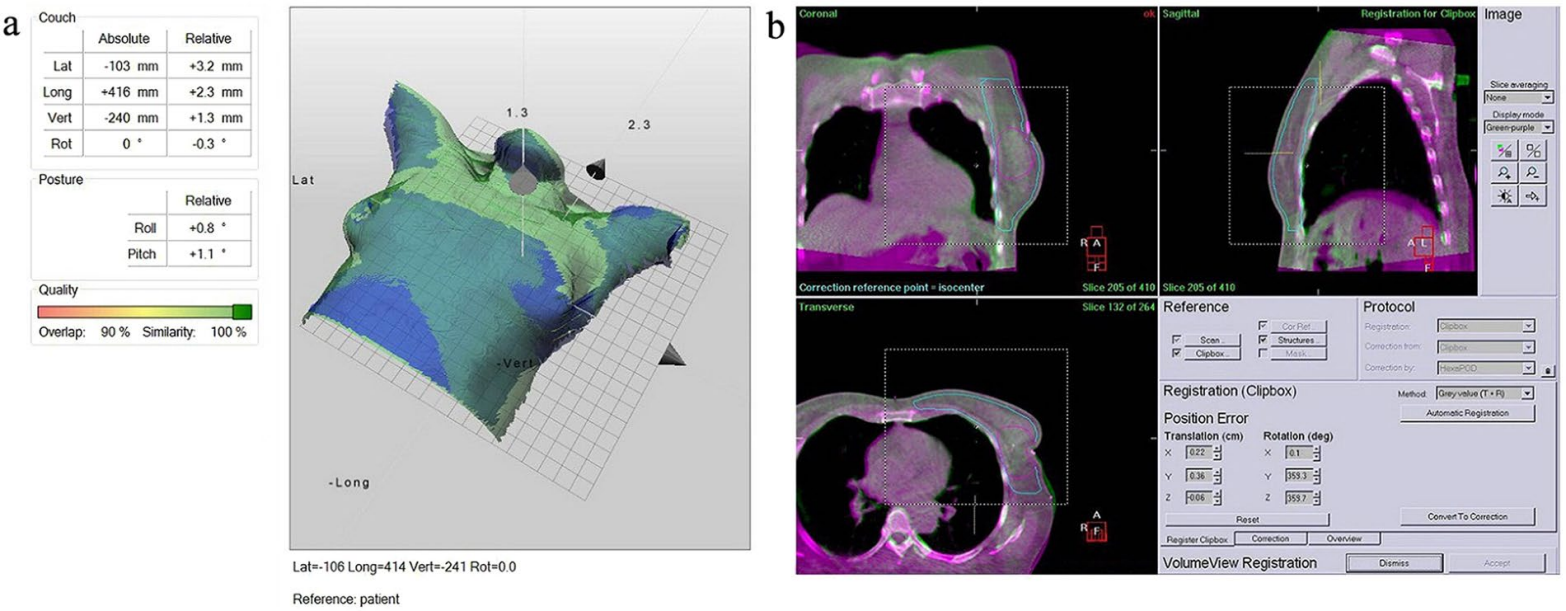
The images and data of setup corrections by Sentinel (a) and CBCT (a,b): The green area represents the body surface profile reconstructed by CT scan, the blue area represents the body surface profile reconstructed by Sentinel scan, and the dark blue represents areas that match with errors. The data on the left is the result of matching error.
